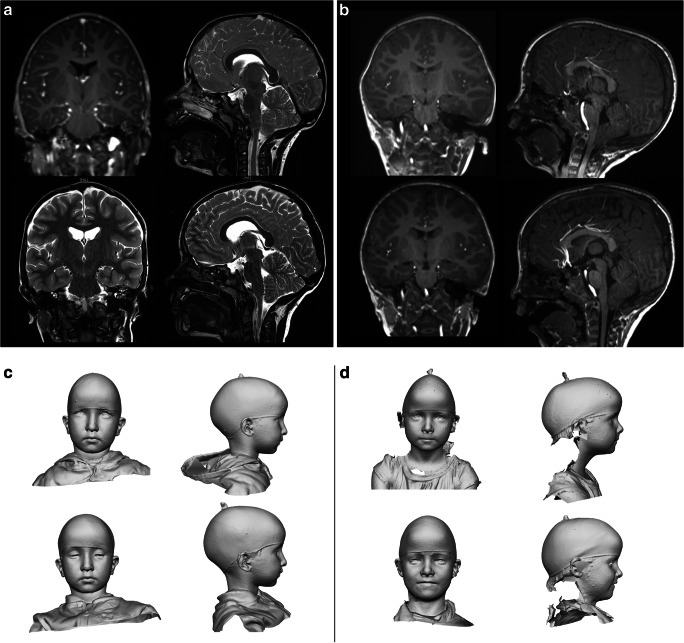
Representative MRI from patients receiving BME surgical procedure for SSS. a 5-year-old boy with sagittal suture synostosis showing biparietal narrowing in the coronal section and tight external CSF spaces in the sagittal section preoperatively (upper left row), which resulted in significant parietal widening and a release of external CSF spaces are shown in the MRI at 5 months after surgery (lower left row). b 3-year-old boy with SSS showing bilateral parietal narrowing in the coronal section and a scaphocephalic descending vertex with secondary Chiari malformation in the sagittal section preoperatively (upper right row). A widened and smooth coronal circumference and elevation of the vertex as well as improvement of the Chiari malformation is shown postoperatively at 9 months after surgery (lower right row). Representative 3D photography from patients receiving BME surgical procedure for SSS. c 6-year-old boy showing a typical scaphocephalic head shape with narrowing in the anterior view and a elongation with parieto-occipital sharpening in the side view preoperatively (upper left row). After surgery, a parietal expansion and a smoother curvature of the convexity are achieved in both views (lower left row). d 5-year-old girl with marked biparietal narrowing in the anterior view and sagittal elongation with decreased vertex slope and secondary frontal bossing preoperatively (upper right row). Postoperatively, the parietal expansion can be visualized in the anterior view and a normalized sagittal curvature with an elevated vertex and less pronounced bossing can be appreciated in the lateral view (lower right row)
